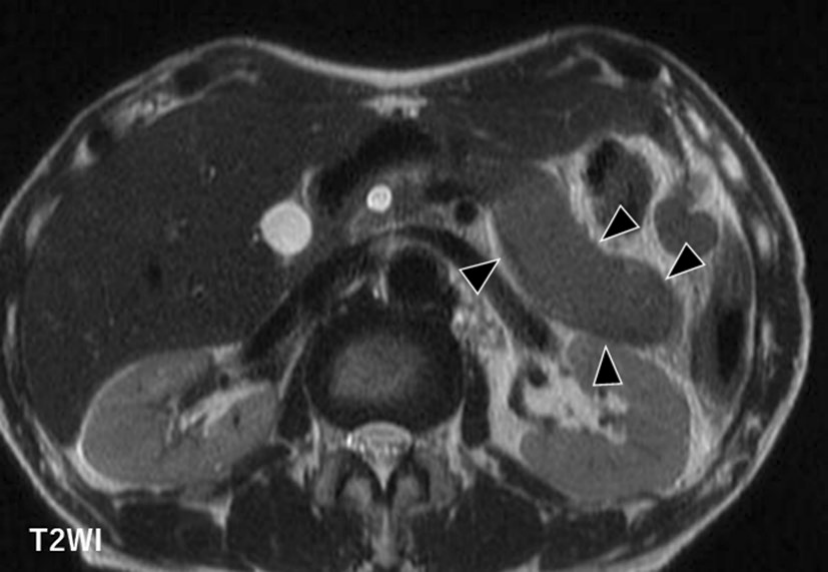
A male patient in his 70 s. On T2-weighted image, autoimmune pancreatitis-affected area shows slightly high intensity demarcated by a low-intensity rim
